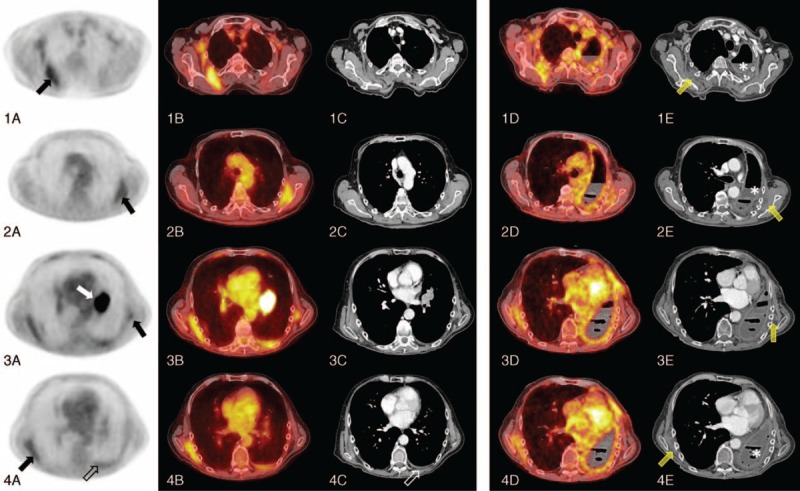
Axial images of an Fluorodeoxyglucose-positron emission tomography-computed tomography (^18^F-FDG-PET) scan performed on a Siemens Biograph mCT PET scanner 60 minutes after administration of 190 MBq ^18^F-FDG (1–4A); axial fusion images of the ^18^F-FDG-PET scan and a free-breathing non-contrast-enhanced computed tomography (1–4B); axial images of a breath hold intravenous iodine contrast computed tomography scan in mediastinal windowing (1–4C). Three-month follow-up axial fusion images of a ^18^F-FDG-PET scan and a free-breathing non-contrast-enhanced computed tomography (1–4D). Three-month follow-up axial images of a breath hold intravenous iodine contrast computed tomography scan in mediastinal windowing (1–4E).
